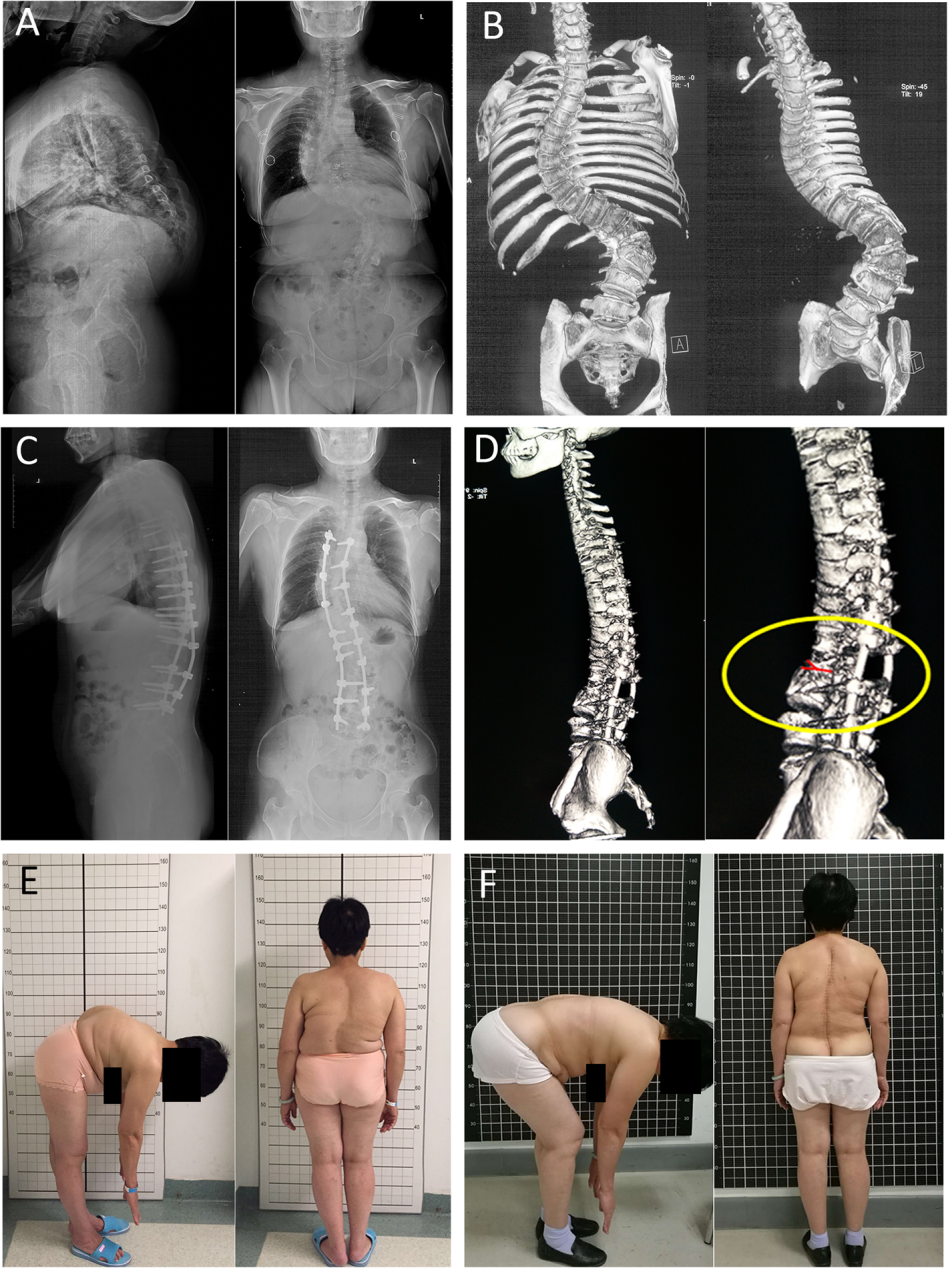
A patient suffering from congenital kyphoscoliosis complained of severe back pain for over 4 years that hardly alleviated with analgesics. a, b Preoperative radiograph and CT scan reconstruction showed a remarkable kyphosis of 90° and scoliosis of 104° in thoracolumbar spine. c Asymmetrical vertebral column decancellation (AVCD) was performed at L2. The sagittal and coronal profile was improved to 25° and 35°as shown in the two-year follow-up images. d The circle in the lower right panel shows the Y-shaped osteotomy. e, f Pre- and Post-operative lateral view shows that the cosmetic disfigurement was improved obviously
